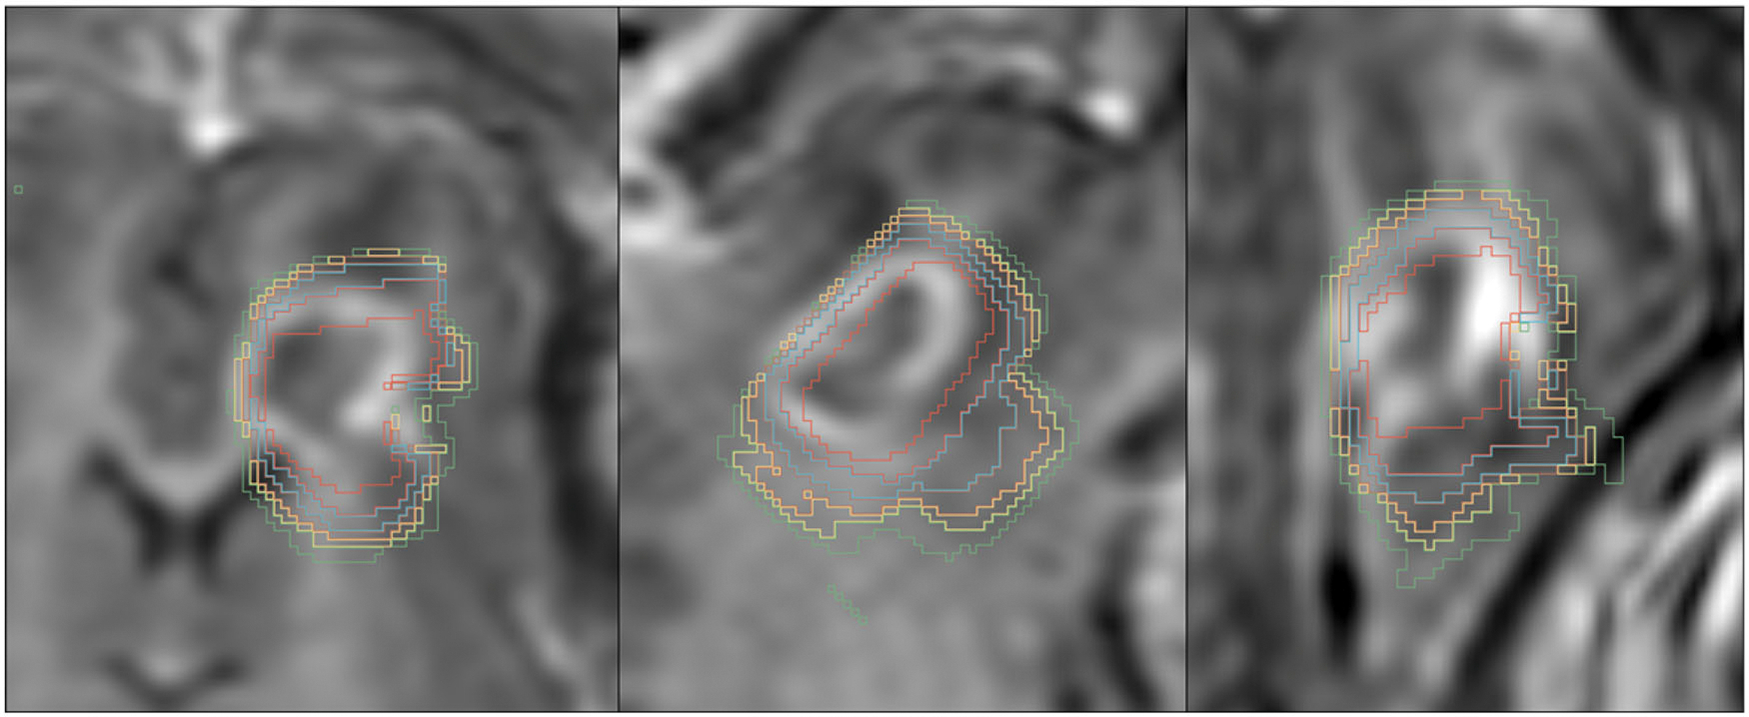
Axial (left), sagittal (middle), and coronal (right) T1-weighted MRI with contrast 2 weeks post-ablation with the 180° probe (3 W, 180 s). The colored zones correspond to 20, 50, 100, 200, 500, and 1000 CEM43 approaching the center of the lesion. These demonstrate that the intermediate- term MRI changes (2 weeks) of the ablation area are in the approximate area of the proximal intraoperative isotherms.
